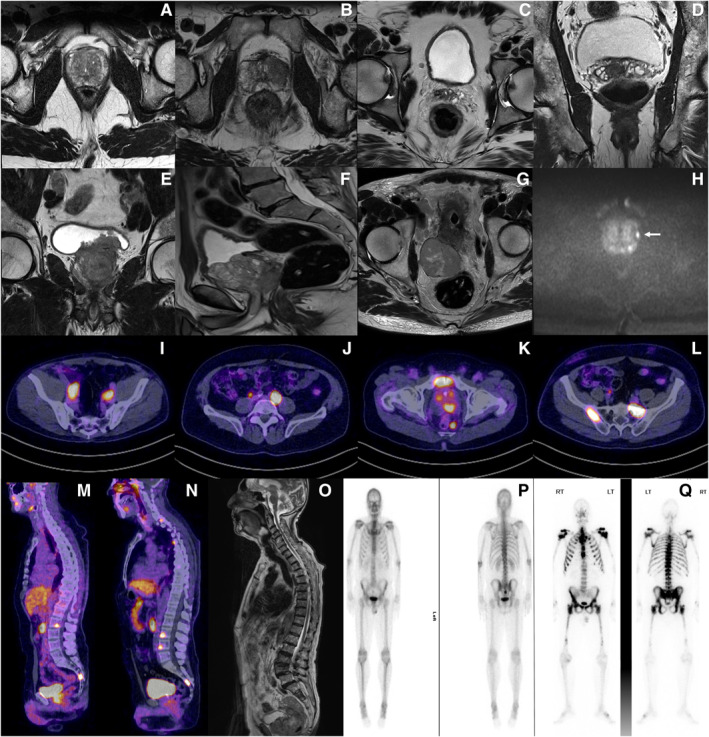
Diagnostic imaging for prostate cancer showing prostate MRI (A‐H), PSMA‐PET (I‐N), whole‐body MRI (O), and bone scans (P, Q). MRI features of organ‐confined disease, (A) T2; capsular bulge and extraprostatic extension, (B) T3a; axial and coronal views of seminal vesicle involvement, (C, D) T3b; adjacent organ involvement, T4, involving (E) urinary bladder, (F) urethra; (G) an exophytic prostatic primary lesion; (H) diffusion‐weighted MRI showing restriction in the left peripheral zone; PSMA‐PET scan showing bilateral pelvic lymph nodes, (I) N1; common iliac lymph node, (J) M1a; mesorectal lymph node, (K) M1a; pelvic bone metastases, (L) M1b; with (M) low‐volume and high‐volume (N) bone metastases; (P) bone scan showing isolated uptake in the sacrum with low‐volume, and (Q) high‐volume bone metastases, *superscan*. Metastatic volume is defined on conventional scans (CT and bone scan) and not PSMA‐PET. CT indicates computed tomography; MRI, magnetic resonance imaging; PET, positron emission tomography; PSMA, prostate‐specific membrane antigen.
